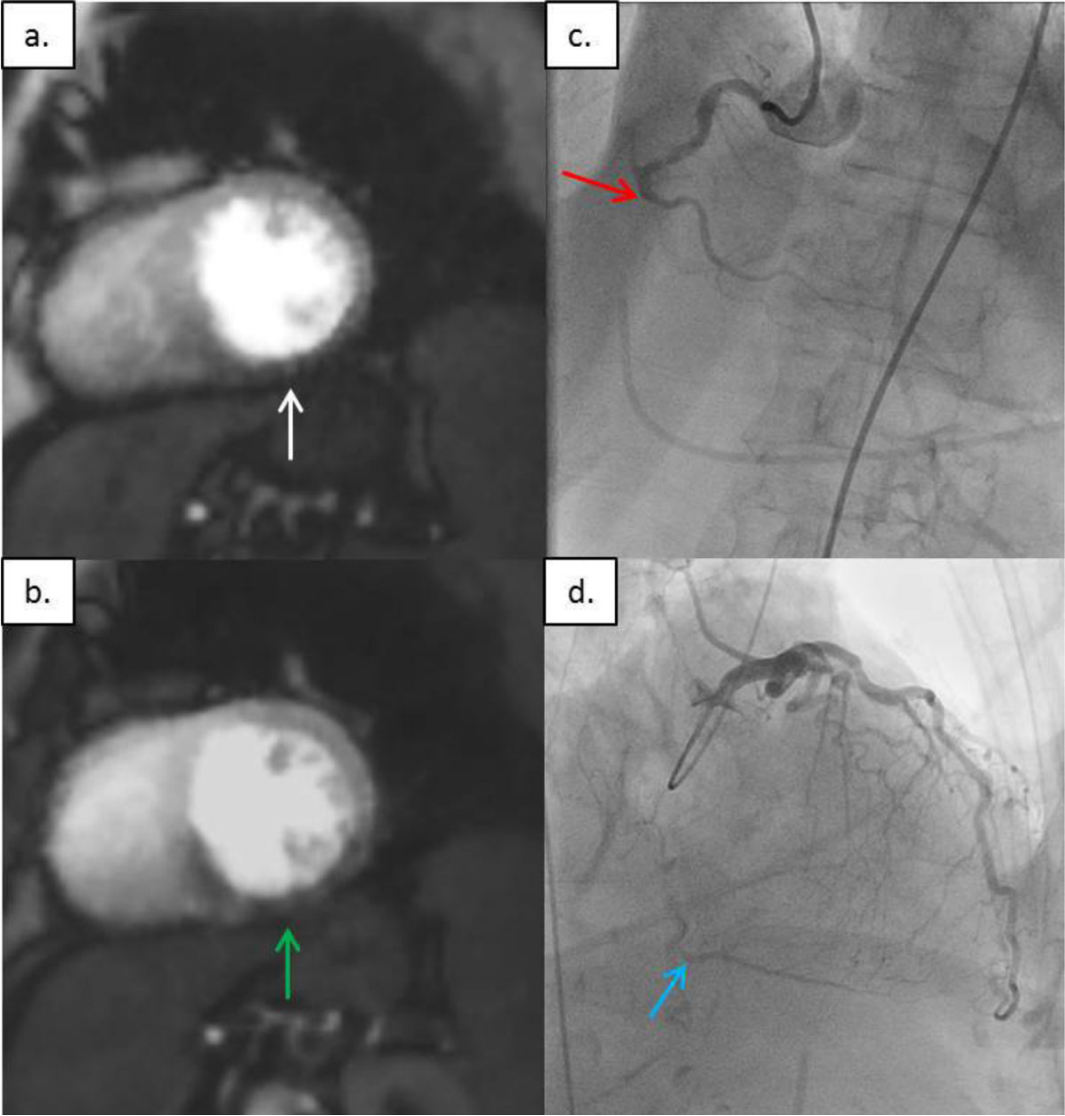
Rest and stress perfusion images showing inferior wall defect with corresponding coronary x-ray angiography. a. Rest perfusion image demonstrating mid inferior wall defect (white arrow). b. Stress perfusion image demonstrating mid inferior wall defect with septal wall extension (green arrow). c. Coronary X-ray angiography demonstrating chronic total occlusion of the mid right coronary artery (red arrow). d. Coronary X-ray angiography demonstrating distal right coronary artery filling via collaterals (blue arrow).
